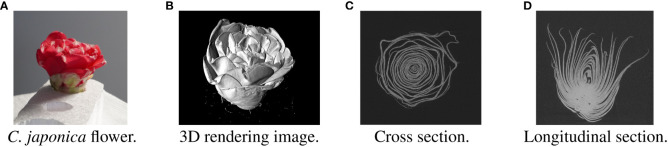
Examples of *Camellia japonica* CT volume images. (A)
